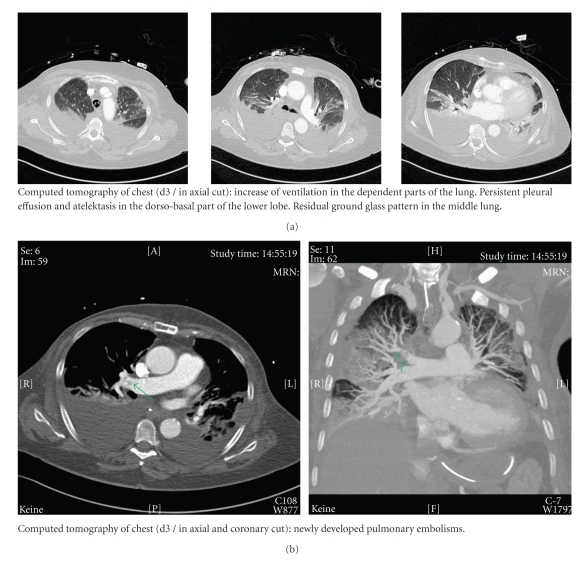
Coronary and axial cuts of the computed tomography of the chest at day 3 after onset of ARDS. d: day; ARDS: acute respiratory distress syndrome.
